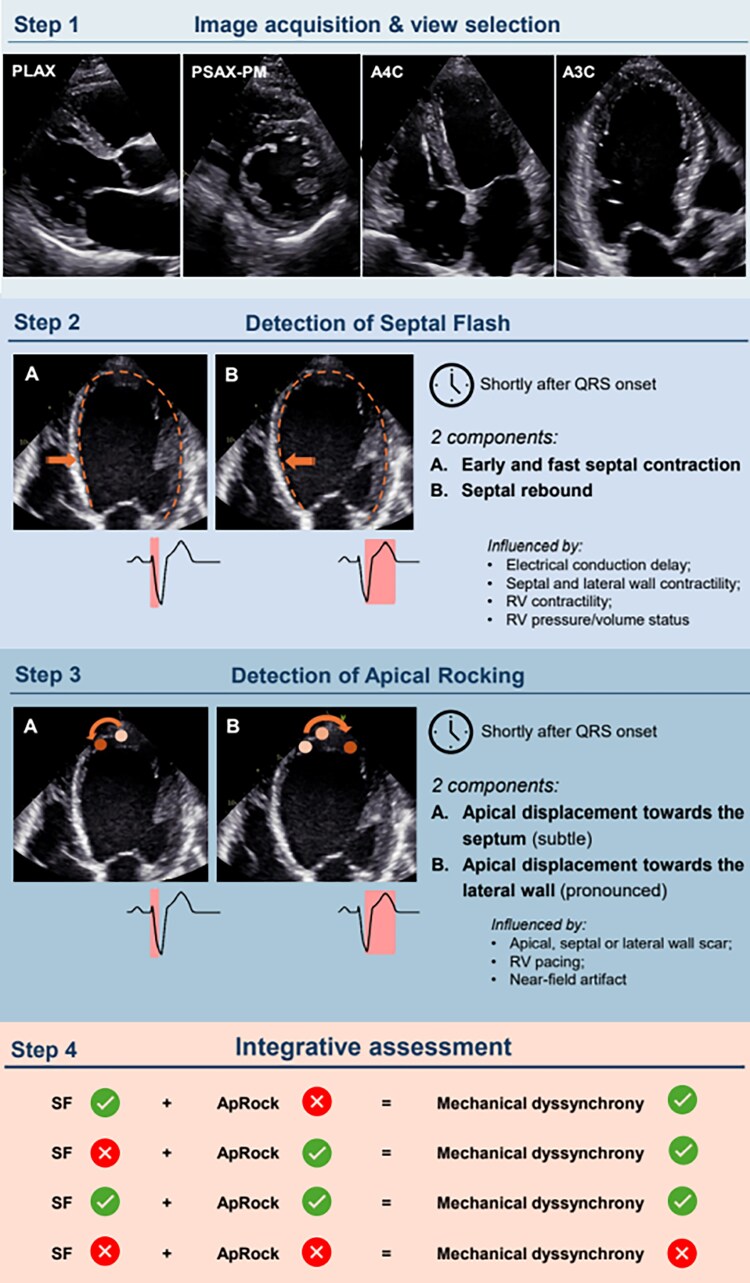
Four steps for diagnosing mechanical dyssynchrony in CRT candidates using echocardiography. A4C, apical 4-chamber view; A3C, apical 3-chamber view; ApRock, apical rocking; PLAX, parasternal long-axis; PSAX, parasternal short-axis; SF, septal flash; RV, right ventricle.
